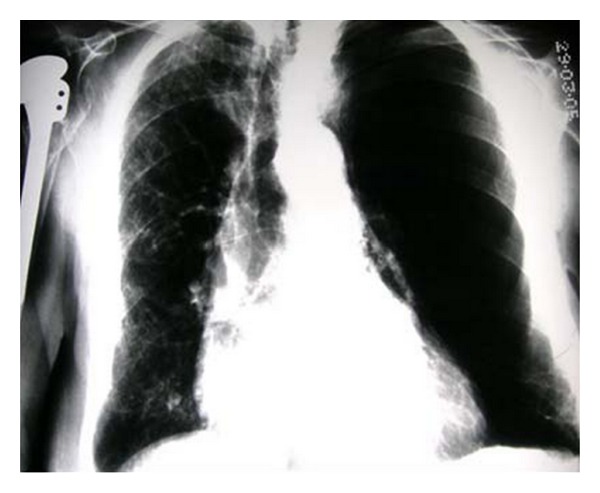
A CXR on a patient with COPD that had undergone a recent shoulder arthroplasty presented with acute shortness of breath. Diagnosis of left-sided pneumothorax was made clinically and from the CXR. A question of bullous emphysema was raised so an urgent CT scan was arranged. See Figure 12.
